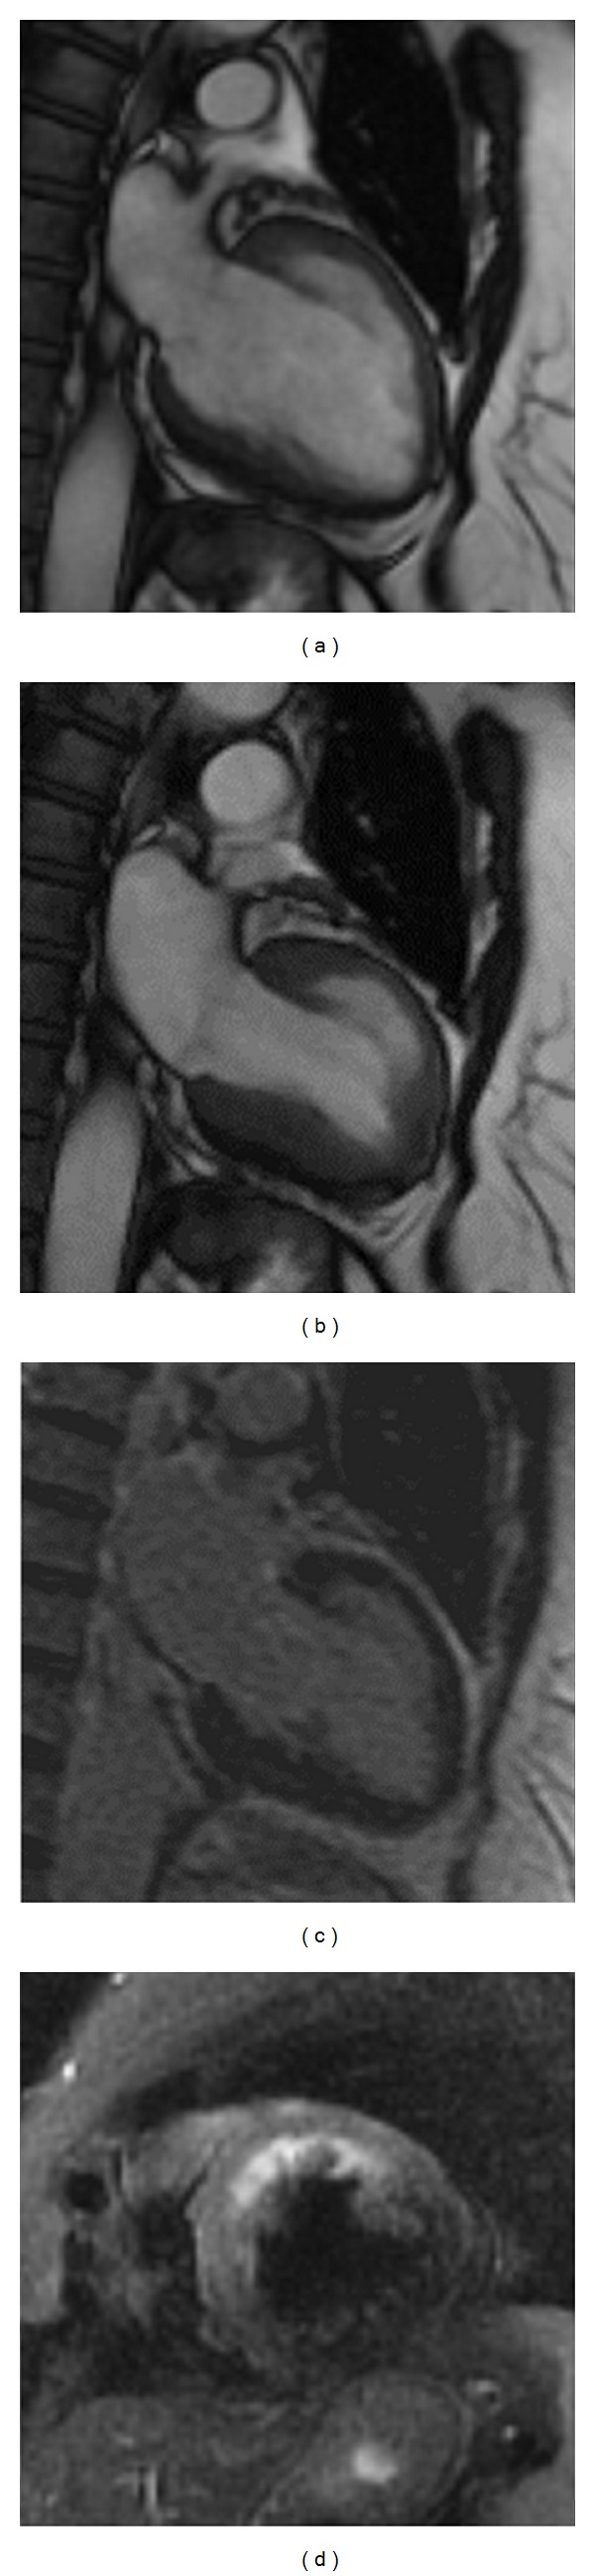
Cardiac magnetic resonance imaging. Enddiastolic and endsystolic 2-chamber view (upper row). Late enhancement 2-chamber view lower left. Anteroseptal edema in the T2 STIR short-axis view (lower right).
